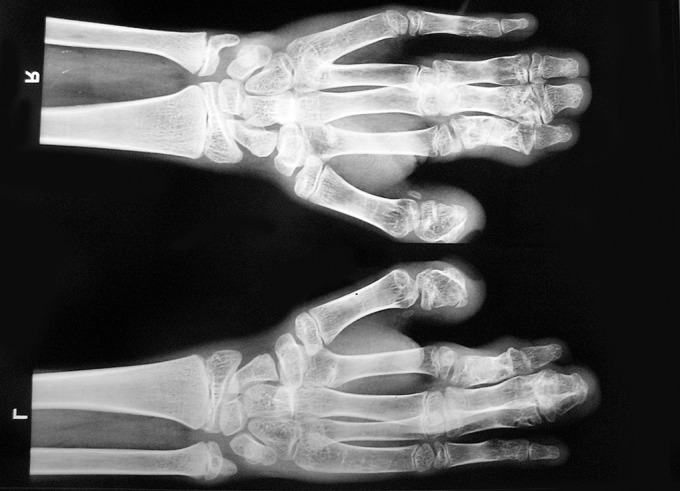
Hands X-ray showing syndactyly of 2nd
